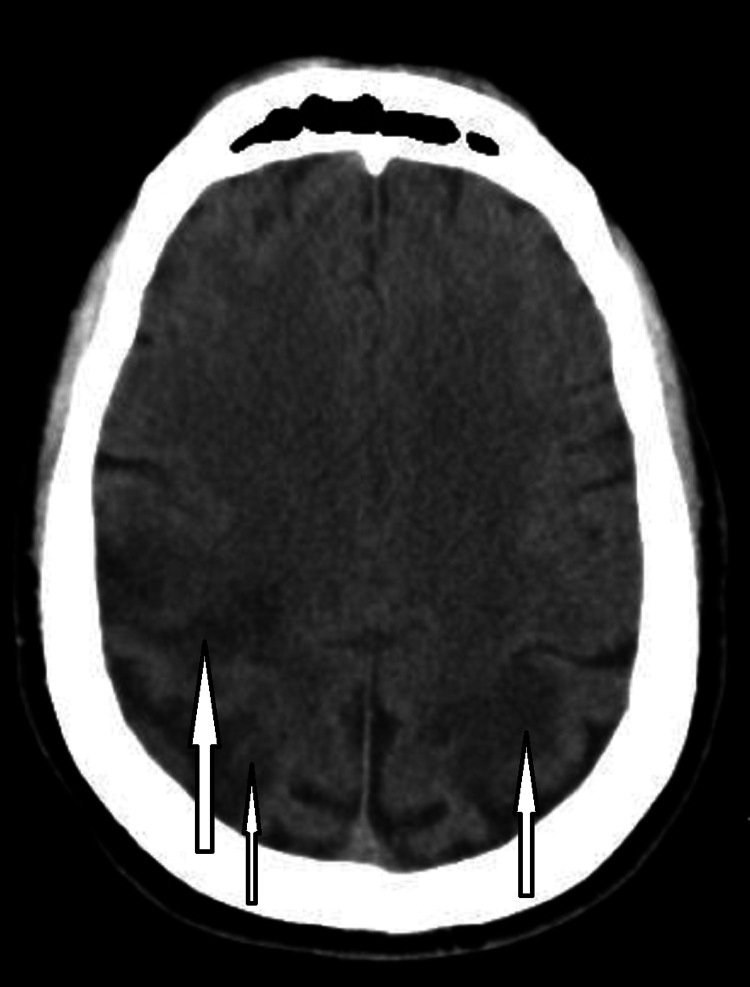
CT of the brain without contrast. The arrows signal hypoattenuation of the parietal and occipital regions. CT = computed tomography
